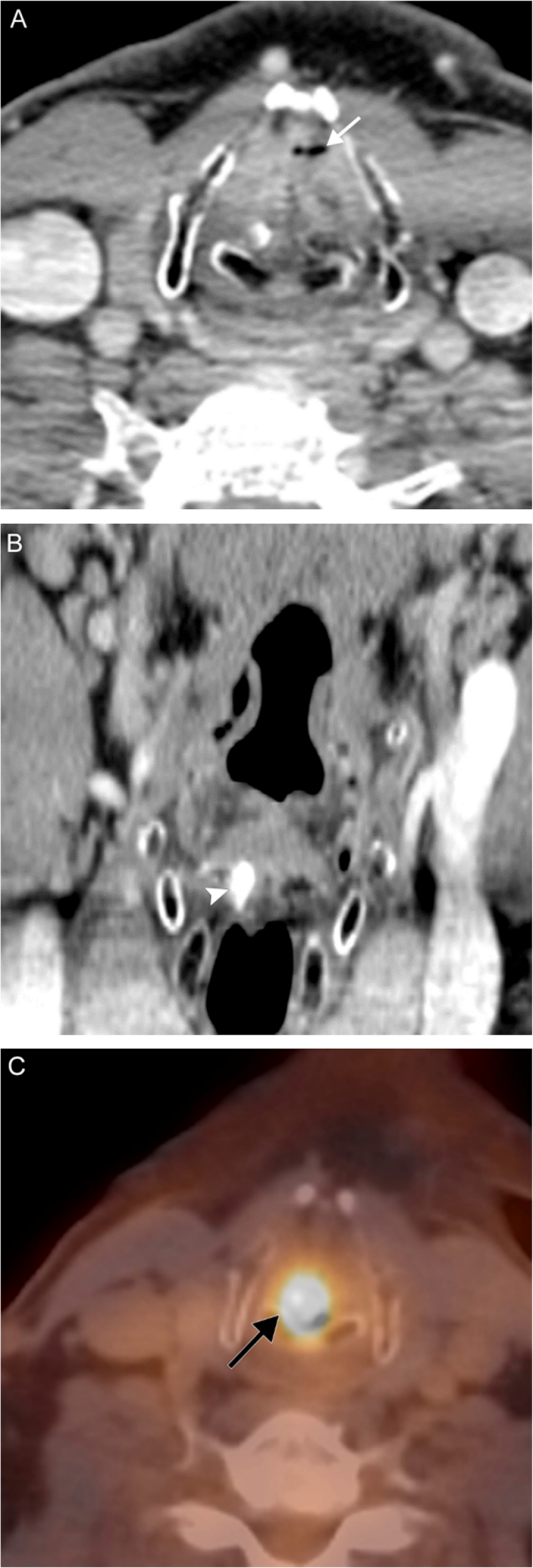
Laryngeal carcinoma. A 59-year-old man with 45-pack-year smoking history presenting with 6 weeks of hoarseness. Axial (a) and coronal (b) contrast-enhanced CT images reveal near-complete obliteration of the laryngeal airway by a polypoid soft tissue mass, which extends inferiorly into the subglottic airway. Note the trace residual airway remnant anteriorly (white arrow). There is asymmetric high attenuation of the right arytenoid cartilage (white arrowhead), consistent with sclerosis and involvement of the lesion. Axial fusion PET/CT image (c) reveals a hypermetabolic mass centered at the larynx (black arrow). Biopsy revealed squamous cell carcinoma
